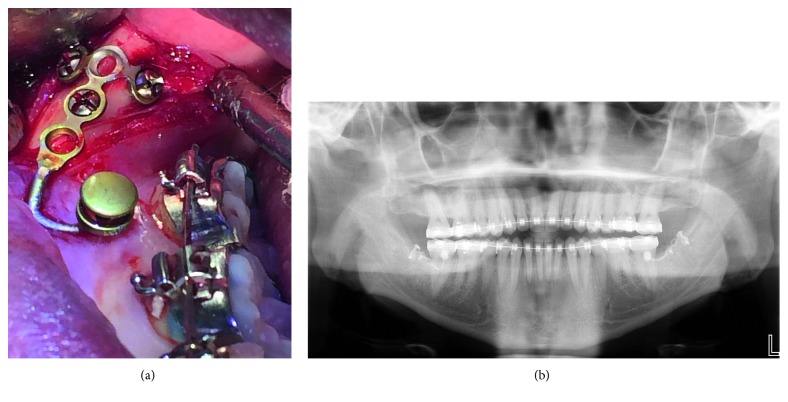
Surgical procedure: (a) intraoral photograph of reflected flap and fixed miniplate; (b) panoramic radiograph after placement of the miniplate.
